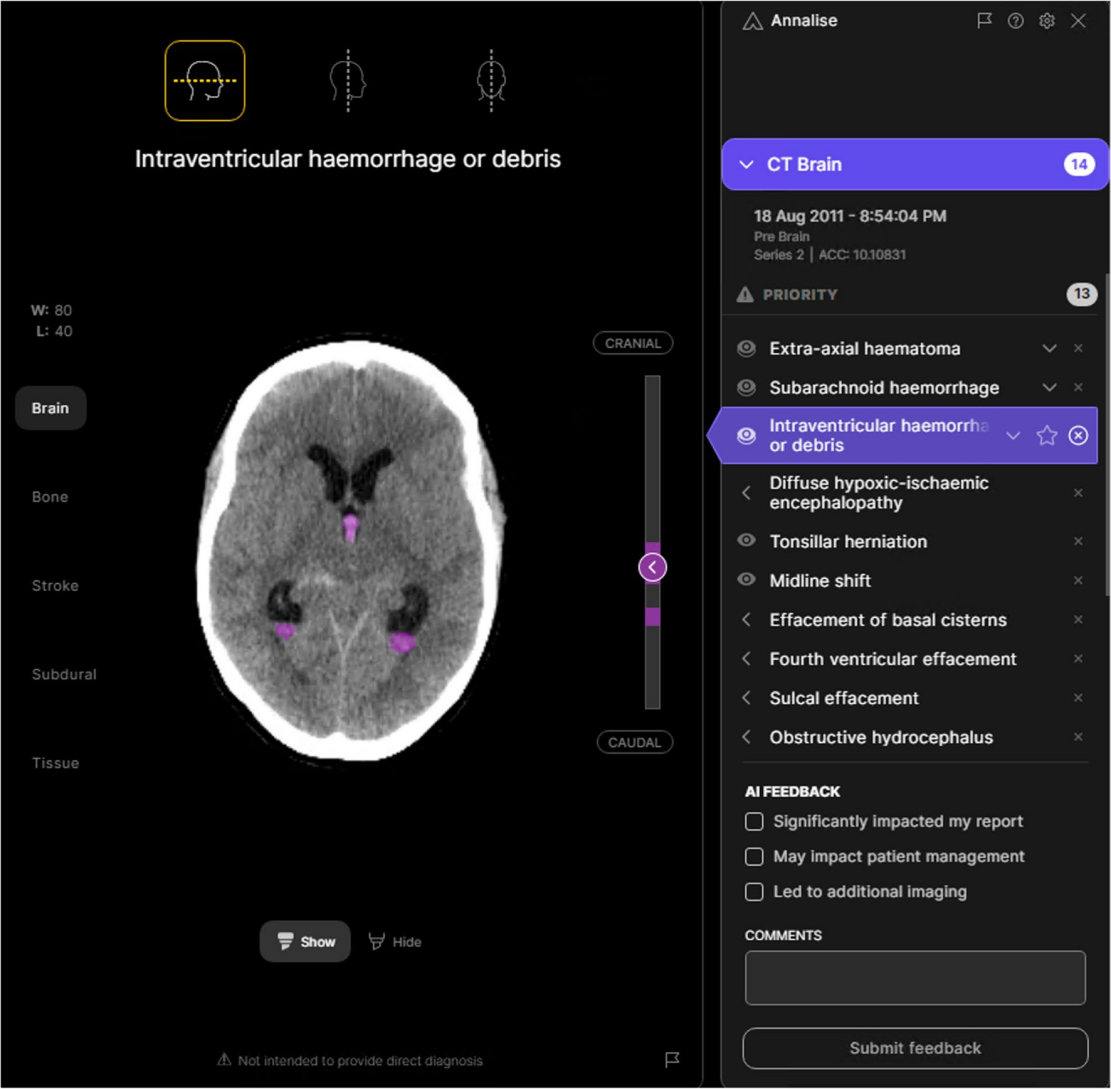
Non-contrast CT brain study demonstrating an intraventricular haemorrhage, along with an example of the decision support system’s user interface
